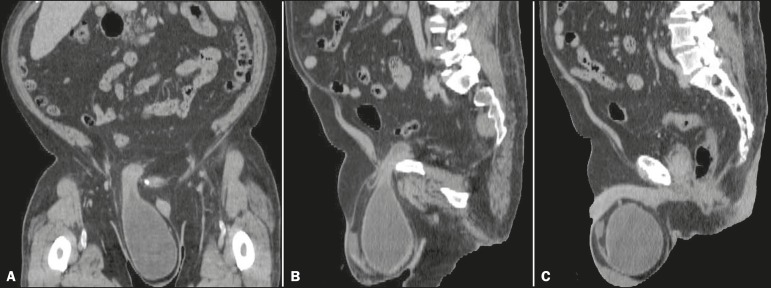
CT images of the abdomen and pelvis, in a coronal view (A) and in sagittal
